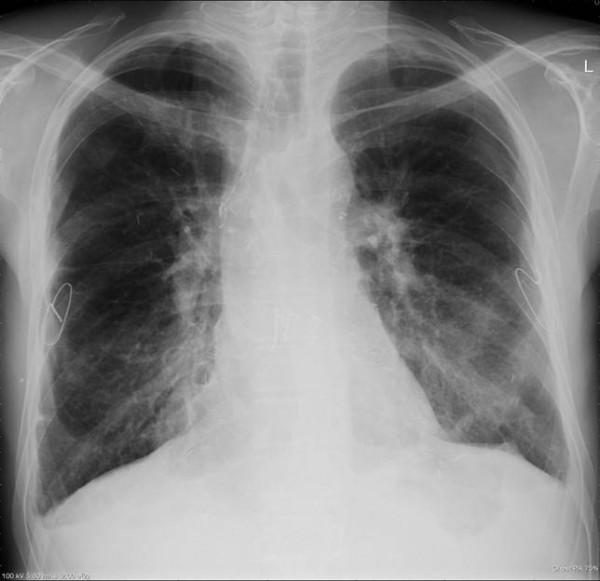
Chest X-ray 4 months following repair of the bronchopleural fistula.
